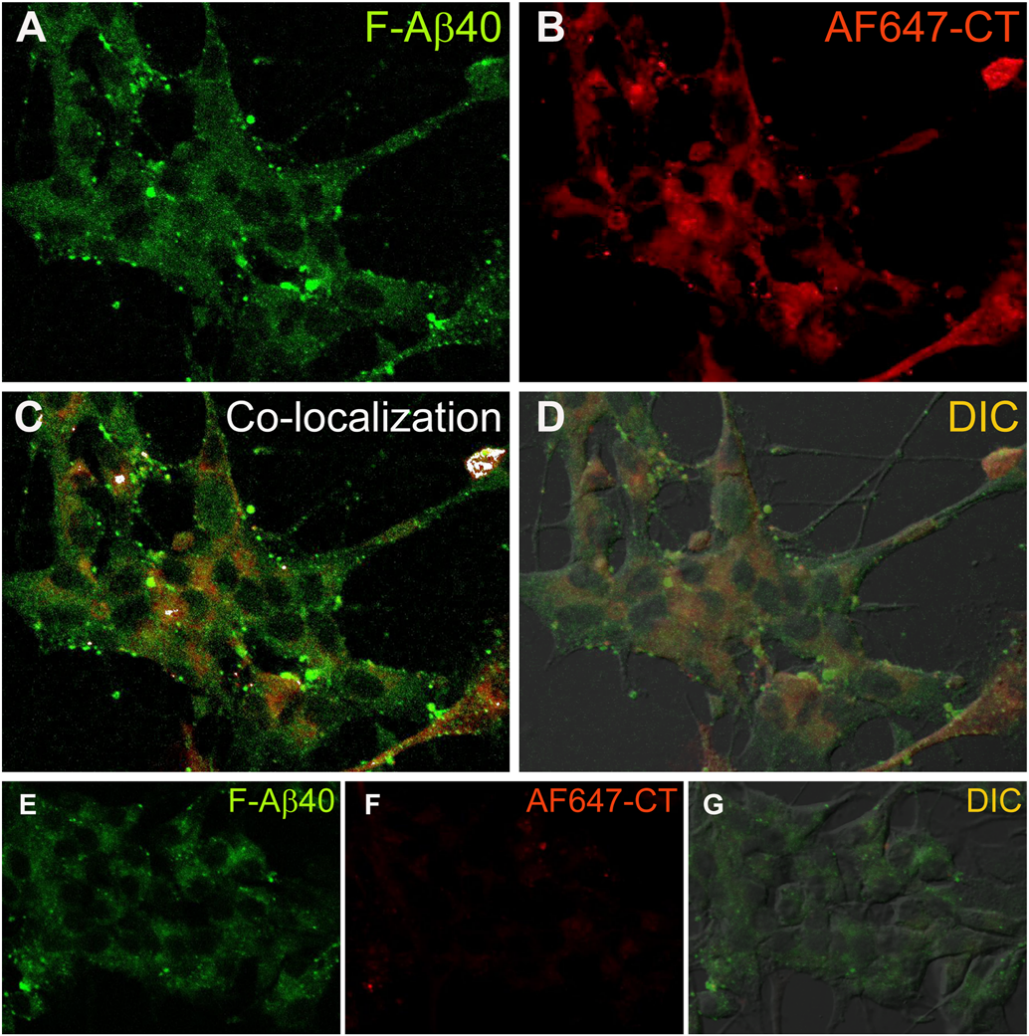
A–D: Uptake of fluorescein labeled Aβ40 (F-Aβ40) and Alexa Fluor® 647 labeled cholera toxin (AF647-CT), caveolae-mediated endocytosis marker, by differentiated PC12 cells following 30 min incubation. (A) F-Aβ40 uptake; (B) Uptake of AF647-CT; (C) Sparse co-localization of F-Aβ40 and AF647-CT as indicated by the white masked areas; (D) Superimposition of images A and B on the differential interference contrast (DIC) image to show the location of the fluorophores in the cells. E–G: Uptake of F-Aβ40 and AF647-CT in differentiated PC12 cells treated with filipin, which is known to inhibit caveolae mediated endocytosis. (E) Uptake of F-Aβ40; (F) Substantial reduction in AF647-CT uptake in the cells treated with filipin; (G). Superimposition of images E and F on the differential interference contrast (DIC) image to show the health of filipin treated cells.
